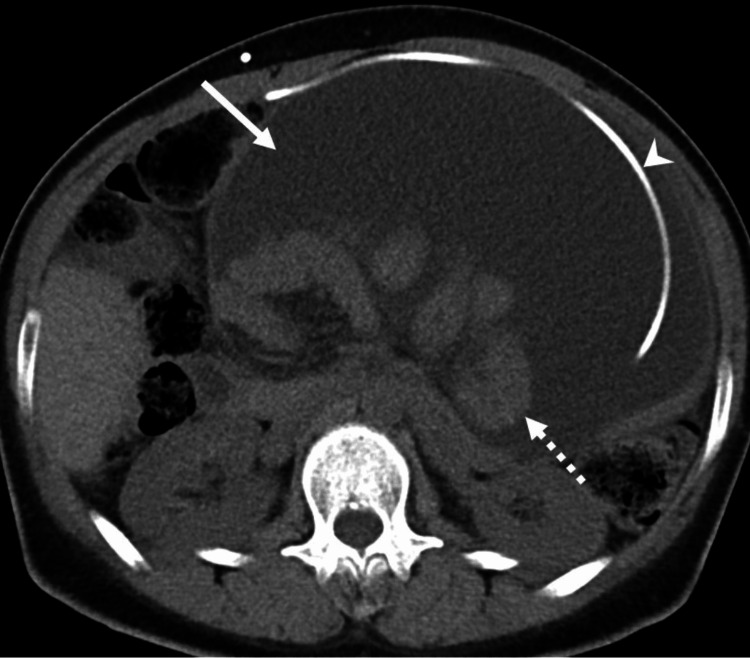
CT scan without contrast CT: computed tomography; VP: ventriculoperitoneal; CSF: cerebrospinal fluid Revealed a large, loculated intraperitoneal fluid collection (solid white arrow) with the VP shunt catheter (white arrowhead) situated within, indicative of a CSF peritoneal pseudocyst. Nondilated small bowel loops were also noted within this collection (dashed arrow)
